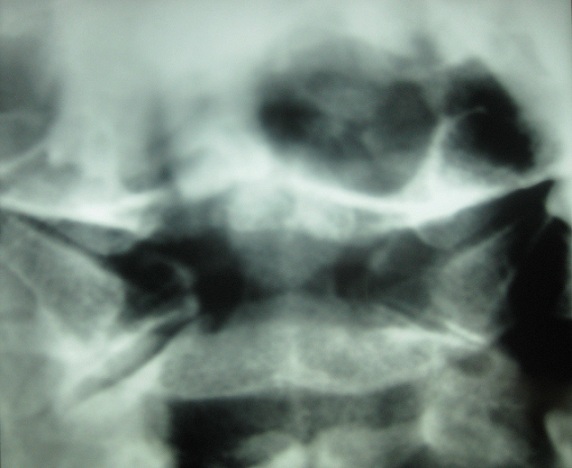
Radiographie du rachis cervical incidence C1-C2 (bouche ouverte): calcifications péri-odontoïdales
